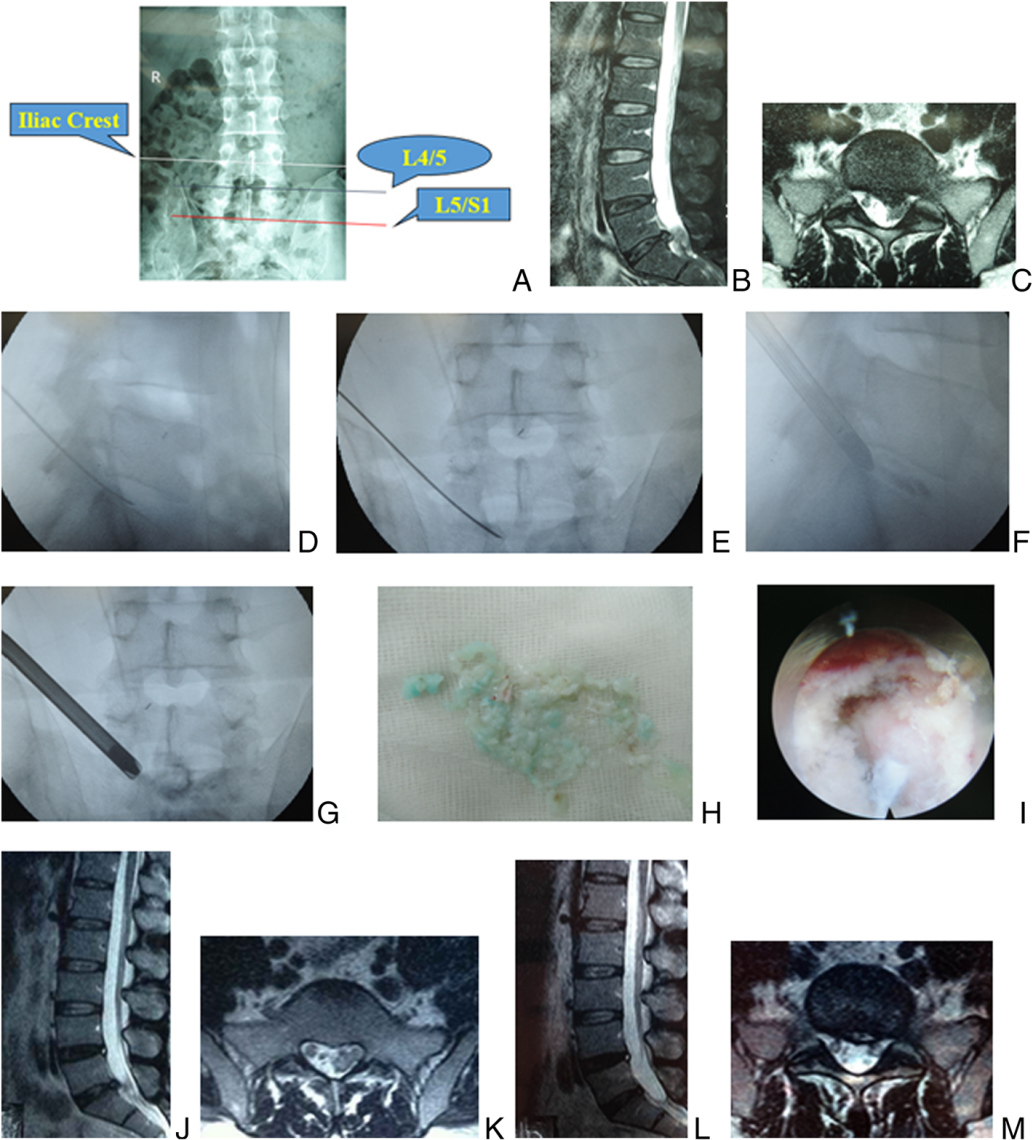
a Posteoanterior X-ray picture, b sagittal, and c axial MR images showed L5/S1 disc herniation with high iliac crest in a 44-year-old man. The tip of the puncture needle was in the posterior one third of intervertebral space on d lateral C-arm view and beyond the medial border of pedicle on e posteoanterior C-arm view. During the procedure of PTES, a 7.5-mm working cannula was advanced over the guiding rod to the vicinity of the sequestrated fragment on f lateral and g posteoanterior C-arm view after the enlargement of the foramen. i Endoscopic picture showed that the nerve root was exposed for complete decompression after removal of h sequestrated disc fragments, which was confirmed on j sagittal and k axial MR images 1 week after operation. After 2 years follow-up, l sagittal and m axial MR images showed no recurrent herniation
